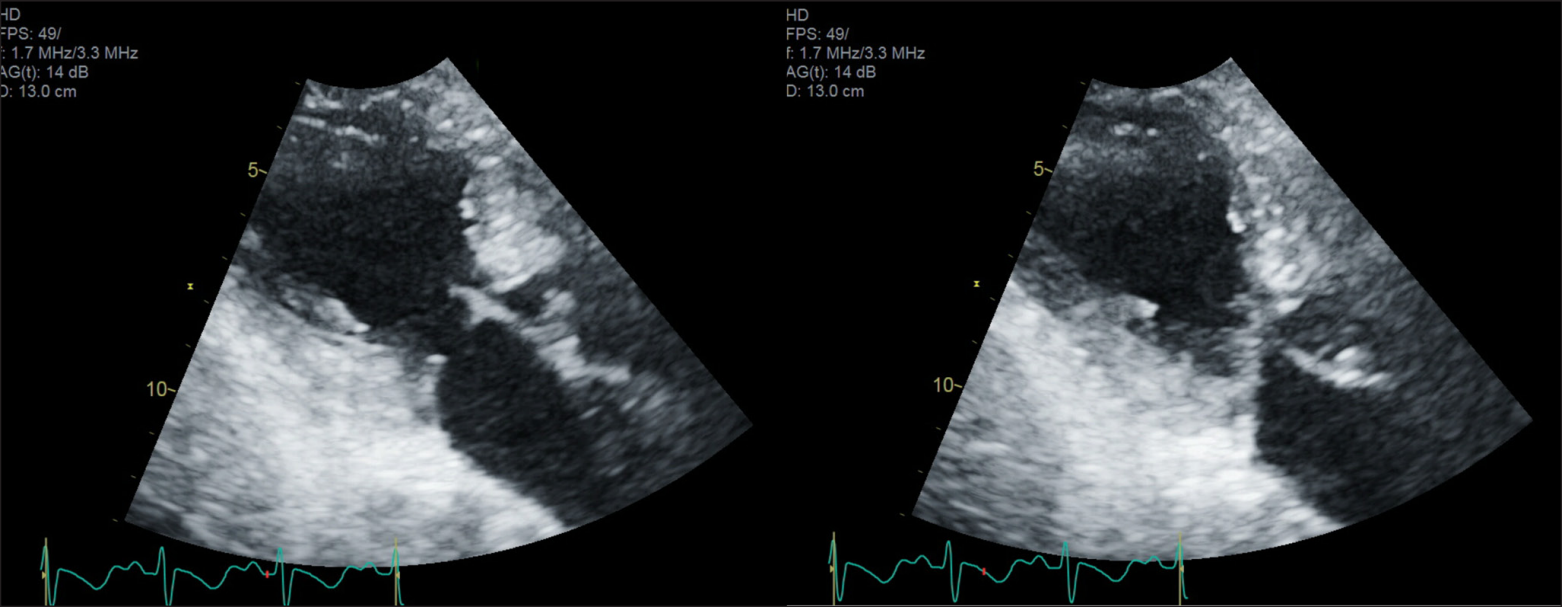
Echocardiogram demonstrated left ventricular outflow tract dynamic obstruction of 85 mm Hg.
